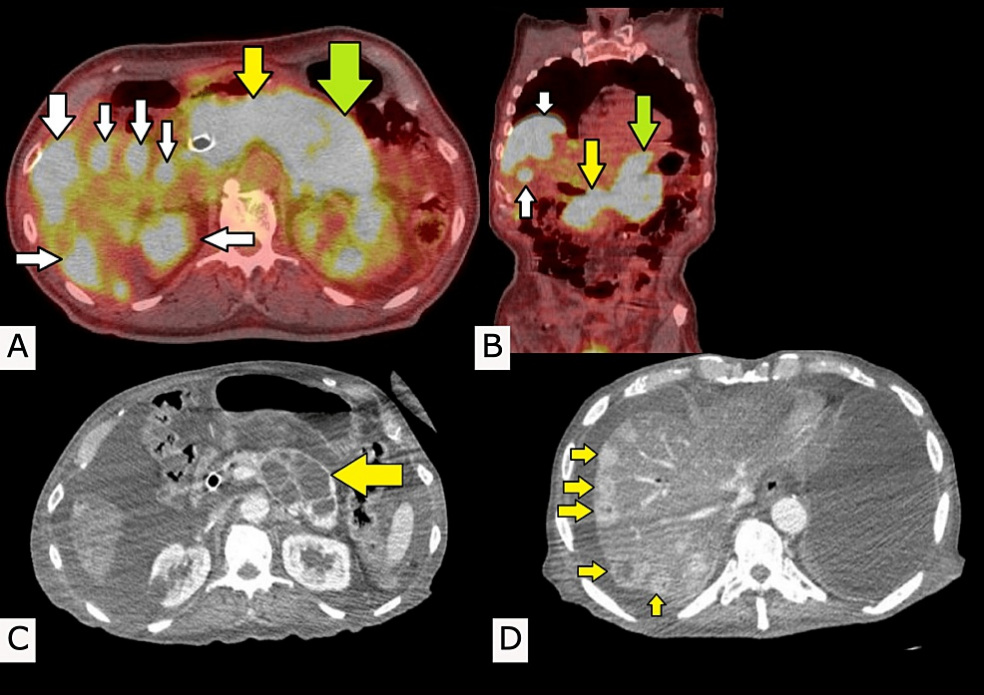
Positron emission tomography (PET) scans and computed tomography (CT) scans (A) Axial and (B) coronal PET scans displaying a large mass involving the body (yellow arrows) and tail (green arrows) of the pancreas with innumerable masses throughout the liver (white arrows). These lesions with considerate metabolism are indicated by hyperlucent (bright white) areas on the scan. (C) Multicystic enlargement of the body and tail of the pancreas (yellow arrow). (D) Multiple enhanced lesions of the liver, some of which are cystic or necrotic in nature (yellow arrows).
